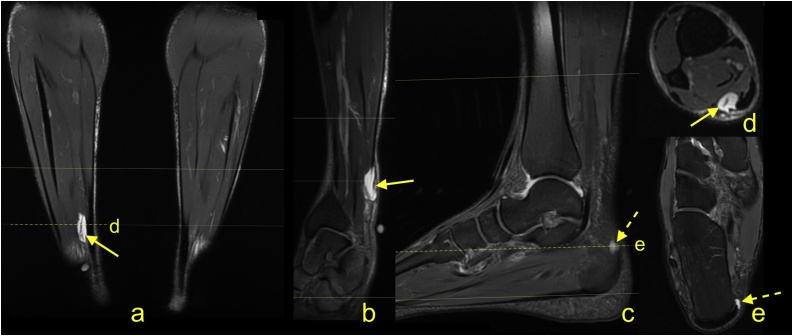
A 27-year-old athlete presents with a palpable focal thickening of the medial part of the Achilles tendon. Clinical suspicion of lipoma. MRI revealed a ganglion-like structure on the medial part of the midportion of the Achilles tendon (straight arrow). A similar lesion is visible on the medial side of the insertion (dashed arrow). No contrast enhancement was noticed, and the radiological diagnosis was ganglions. Surgery was performed, and the proximal lesion was removed. The postoperative diagnosis was ganglion. a-e - PD-weighted with fat suppression.
